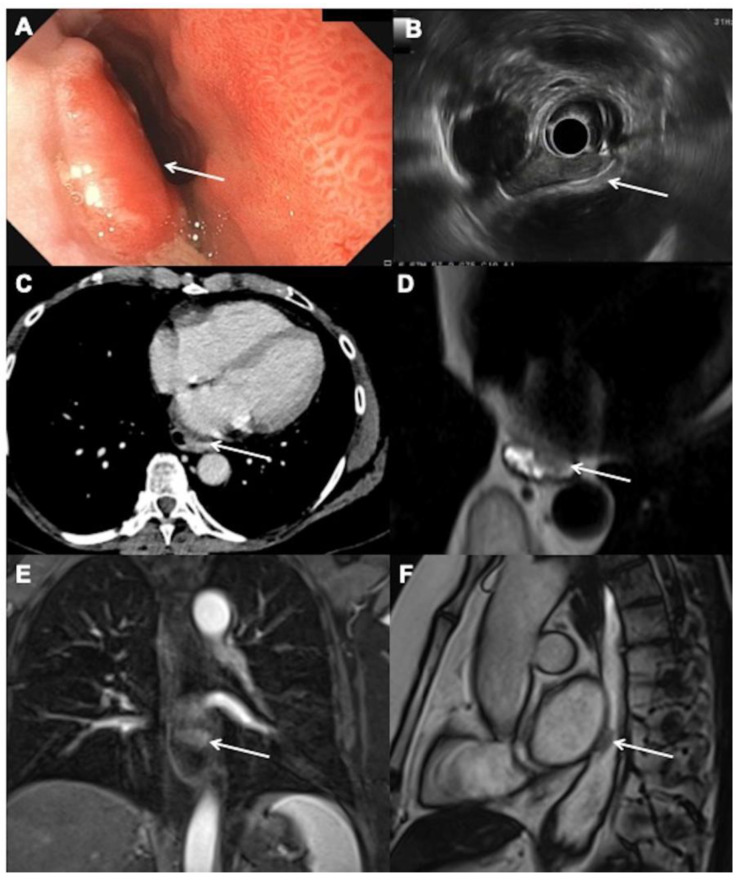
Initial staging for a 66-year-old woman with T2N0M0 esophageal adenocarcinoma: (A) endoscopic view of an elevated neoplastic lesion arising on a Barrett’s esophagus on the left posterior side of the esophagus, 34 cm from the dental arch (arrow). (B) Endoscopic ultrasound showing the hypoechoic, well-limited neoplastic lesion in close contact with the muscularis propria without regional lymph nodes (arrow). (C) Contrast-enhanced computed tomography image obtained at 70 s after intravenous administration of iodinated contrast material and without oral contract material in the axial plane shows irregular esophagus (arrow) but no definite lesion. (D) Axial T2-weighted single shot magnetic resonance (MR) images. after oral administration of water show a low signal irregular anterior lesion (arrow). (E) Coronal contrast-enhanced T1 weighted images confirm the lesion that demonstrates an early contrast uptake (arrow). (F) Sagittal steady-state MR images confirm the low signal lesion (arrow) and allow to precisely see its location.
